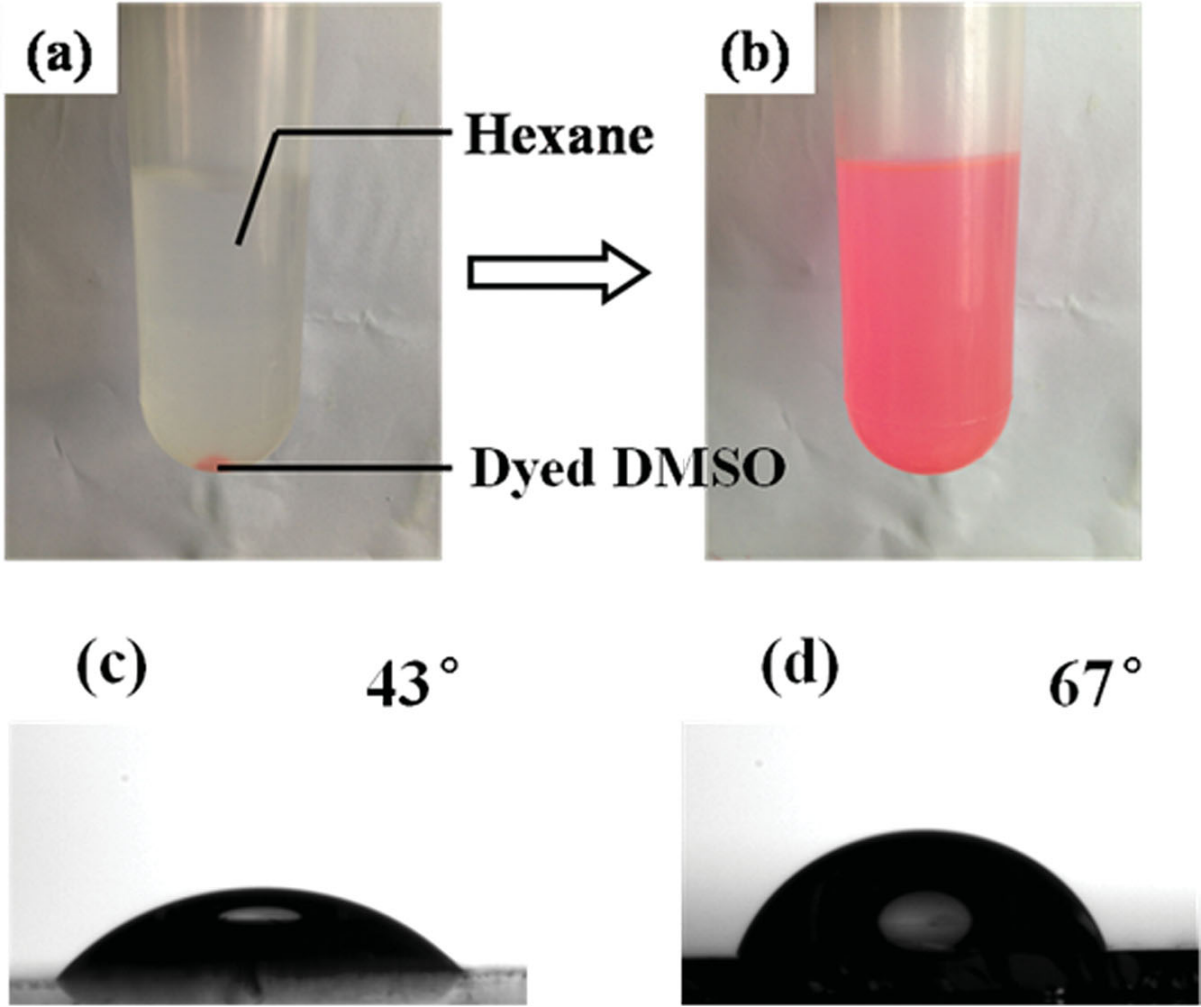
Photographs of Rhodamine 6G dyed DMSO/hexane mixture a) before and b) after ultrasonication, and contact angle of DMSO on c) Au and d) OTS modified Si.
